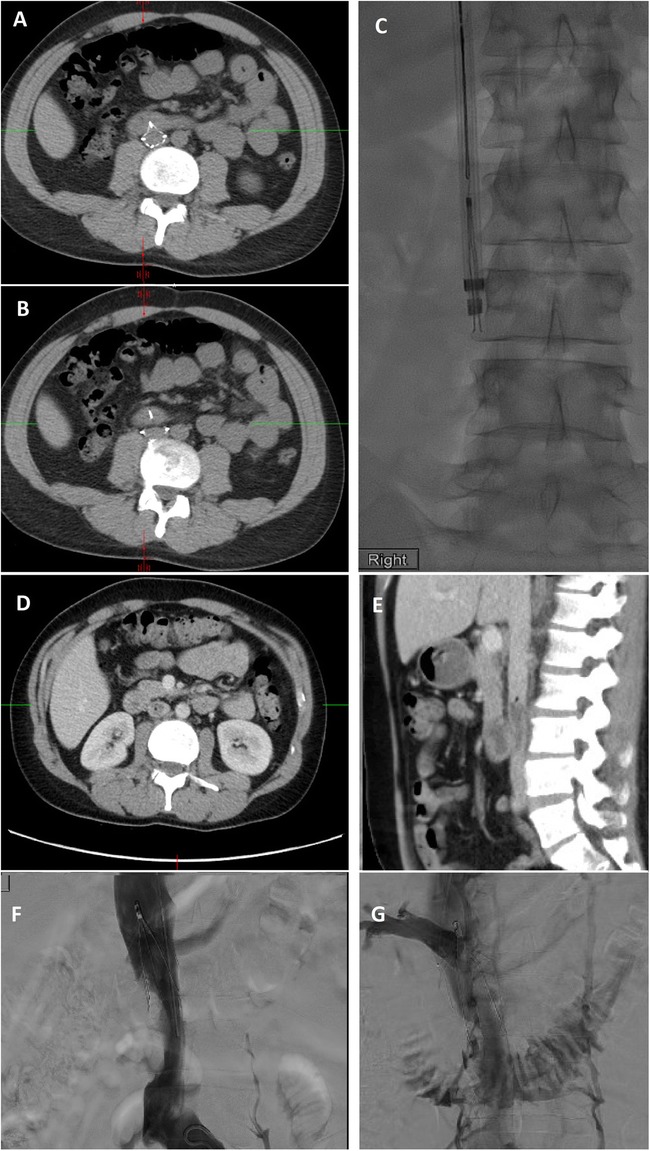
This patient had a Günther Tulip IVC filter (Cook, Bloomington, IN) for PE prophylaxis after a motor vehicle accident and was lost to follow-up until imaging was done for unrelated reasons 10.5 years later. Pre-retrieval CT (a + b) showed penetration of 2 legs of the filter into the doudenum. Given embedded legs, a a 14Fr Excimer laser sheath was employed (c). The day after retrieval, the patient developed fevers and 8 days later he underwent CT of the chest, abdomen and pelvis. Chest CT showed multiple cavitary nodules, pulmonary emboli (not shown) and new IVC clot with a small focus of gas at the cranial aspect of the clot (d + e). After filter placement cranial to this clot (f) and subsequent clot aspiration 20 French FlowTriever® Aspiration device (Inari Medical, Irvine, CA), cavagram showed a fistula to the doudenum (g). After the patient was placed on antibiotics and anticoagulation, bacteremia and IVC clot resolved. A case of a doudenal-caval fistula caused by an in-situ IVC filter has been reported in the literature (Vandy et al. 2011). Doudenal-caval fistula or septic VTE have not yet been described after filter removal, however
